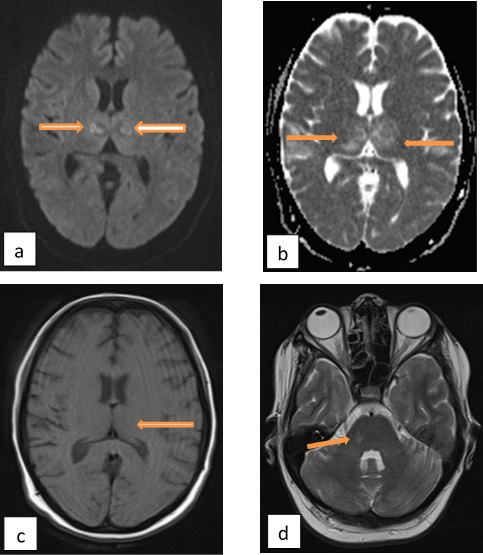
Final MRI brain was done before the discharge of the patient. (a) axial DWI and (b) axial ADC map with remarkable regression of the diffusion restriction at thalami. (c) axial *T*_1_WI at the level of thalami with the disappearance of the hemorrhagic areas. (d) axial *T*_2_WI at the posterior fossa shows disappearance of edema at the cerebellum with a marked decrease in size of edematous area at the pons. ADC, apparent diffusion coefficient; DWI, diffusion-weighted imaging
